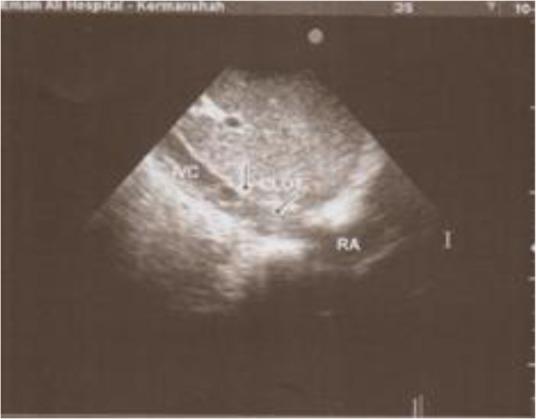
Echocardiography shows thrombosis in inferior vena cava extended to right atrium
